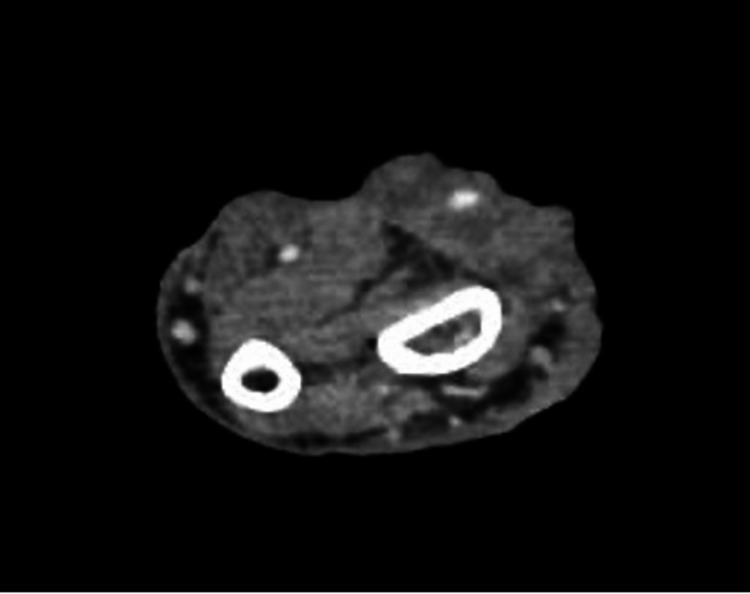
CT imaging of Case 3 prior to vismodegib treatment, demonstrating encasement of the radial artery.
